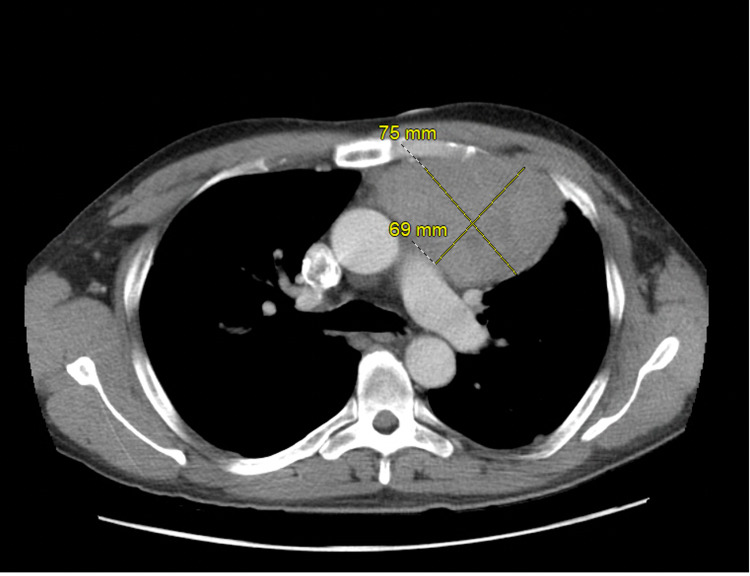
Chest-CT with contrast, axial view
